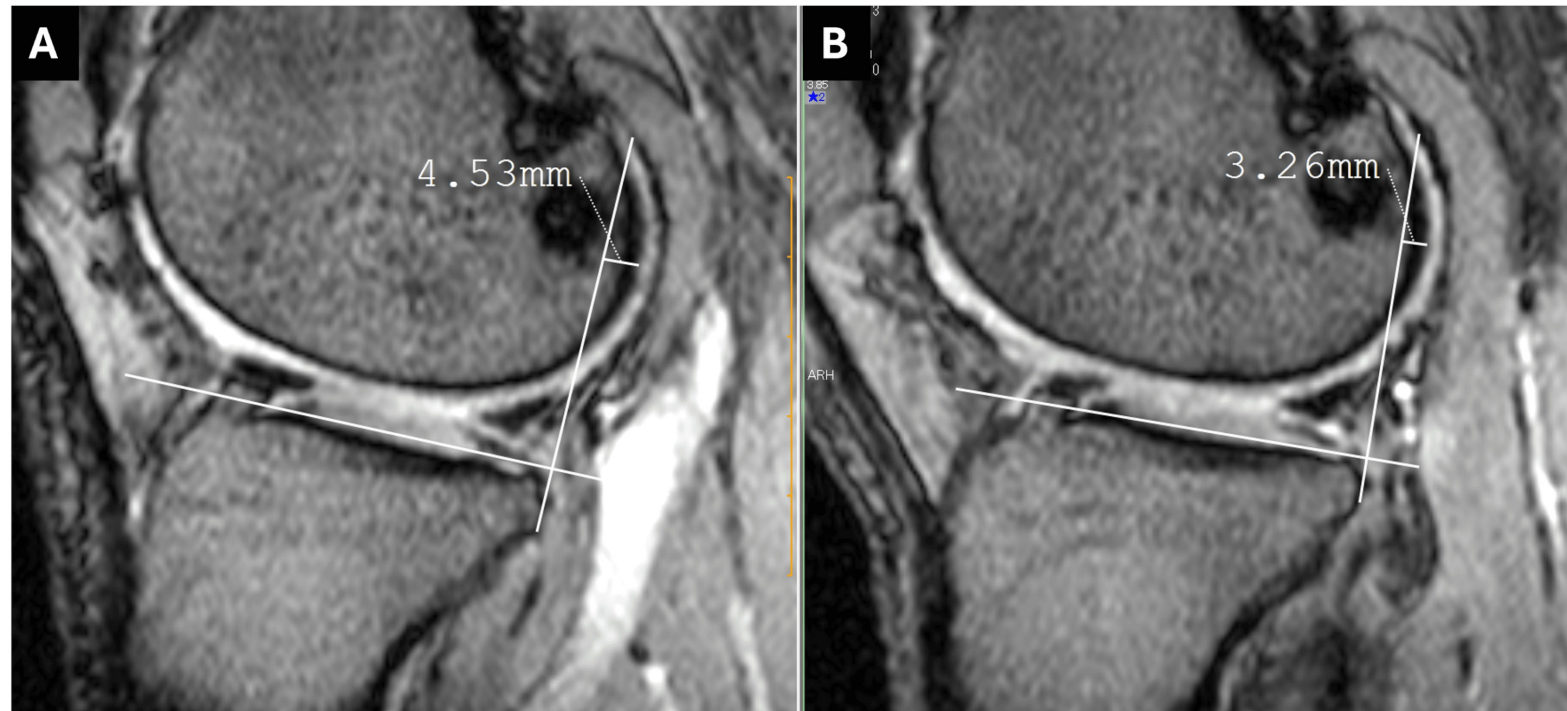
MRI showed that the distance between the rearmost part of the lateral femoral condyle and the posterior edge of the lateral tibial plateau was 1.27 mm shorter after arthroscopic surgery: (A) before arthroscopic surgery and (B) one year after arthroscopic surgery. MRI: magnetic resonance imaging
